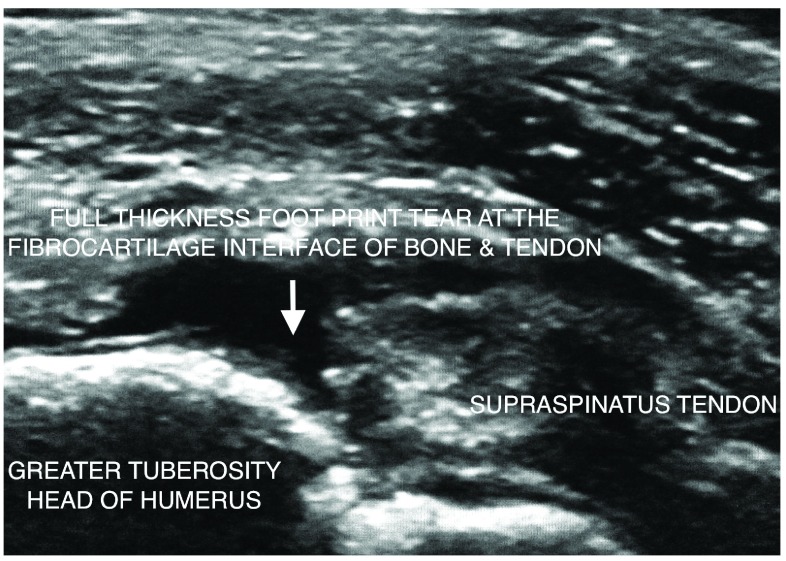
Long axis ultrasound image shows a full thickness footprint supraspinatus tear superimposed on degenerative tendinosis. Tear outline is well appreciated from distension of the peritendinous and subdeltoid bursal space during percutaneous treatment with tenotomy and liquid platelet rich plasma.
